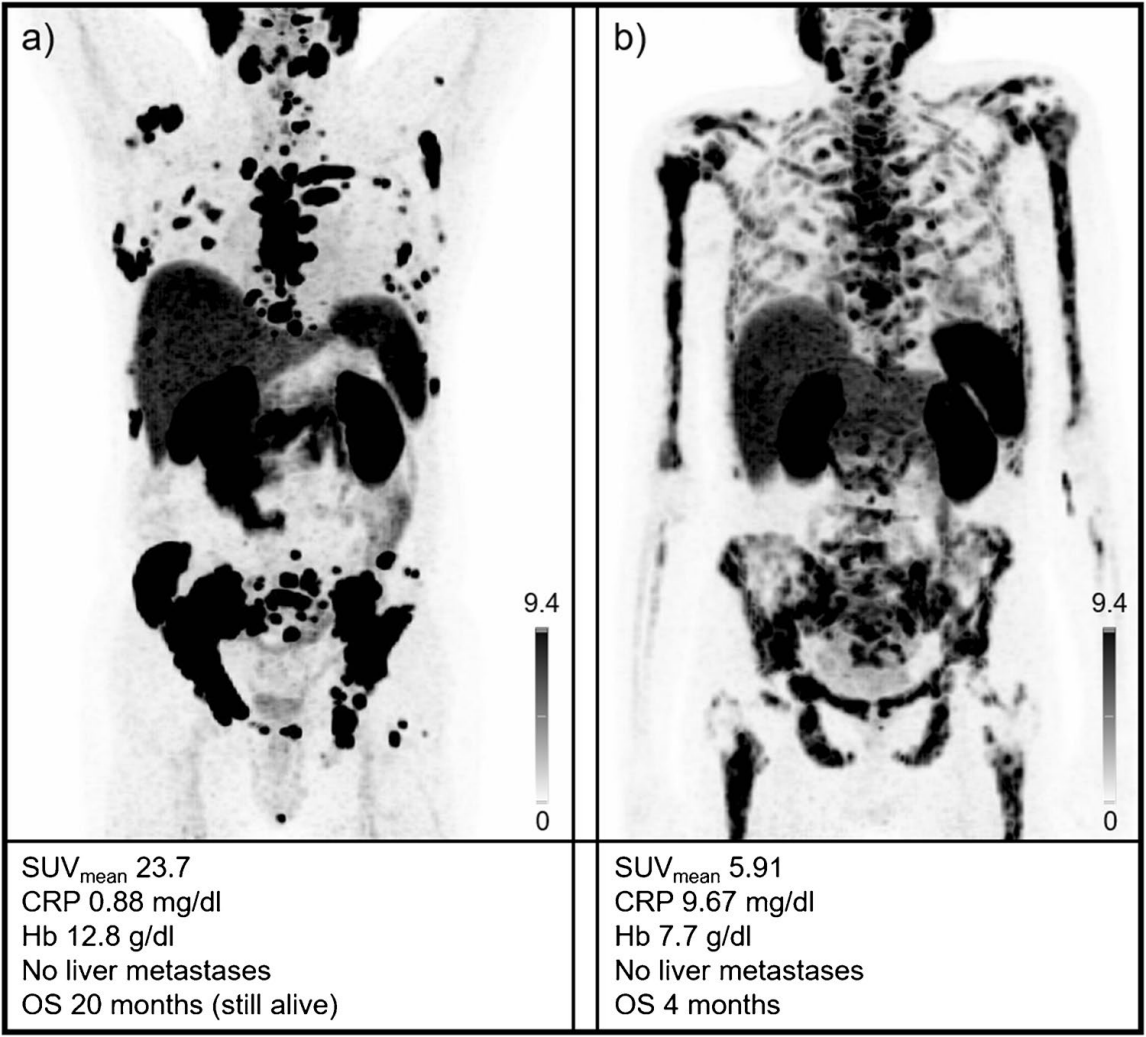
Baseline [^18^F]PSMA-1007 PET of two patients with different outcomes after radioligand therapy with [^177^Lu]Lu-PSMA I&T. Maximum intensity projections are displayed. The baseline values of the different parameters and the maximum intensity projections are also provided. a This patient showed high tracer uptake and no risk factors apart from slightly elevated CRP levels. b This patient exhibited only moderate tracer uptake with a patchy pattern, elevated CRP and reduced hemoglobin, resulting in three risk factors. Overall survival of the patient provided in (a) was significantly longer (20 months at date of censoring versus patient in (b) with 4 months). SUV = standardized uptake value, CRP = C-reactive protein, Hb = hemoglobin, OS = overall survival
